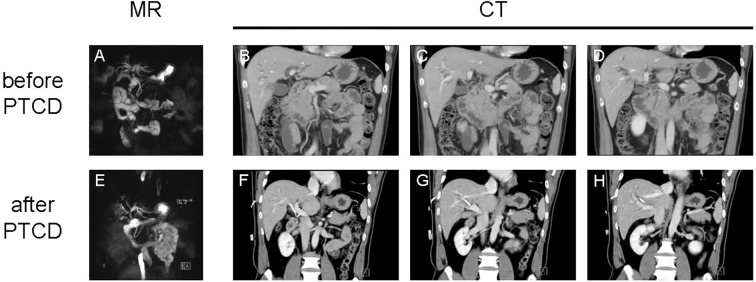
Radiological evaluation of the patient before and after percutaneous transhepatic cholangial drainage (PTCD). (A) Reconstructed MRCP image prior to PTCD demonstrating high biliary obstruction. (B–D) Representative contrast-enhanced CT images before PTCD showing a slightly low-density mass at the hepatic hilum with slightly thickened bile duct walls and lumen narrowing (red arrowheads indicate the enhanced mass and thickened walls). (E) Reconstructed MRCP image after PTCD showing the drainage tube and alleviated intrahepatic duct dilation. (F–H) Post-PTCD contrast-enhanced CT images demonstrating the stable, persistently enhanced mass at the hepatic hilum.
